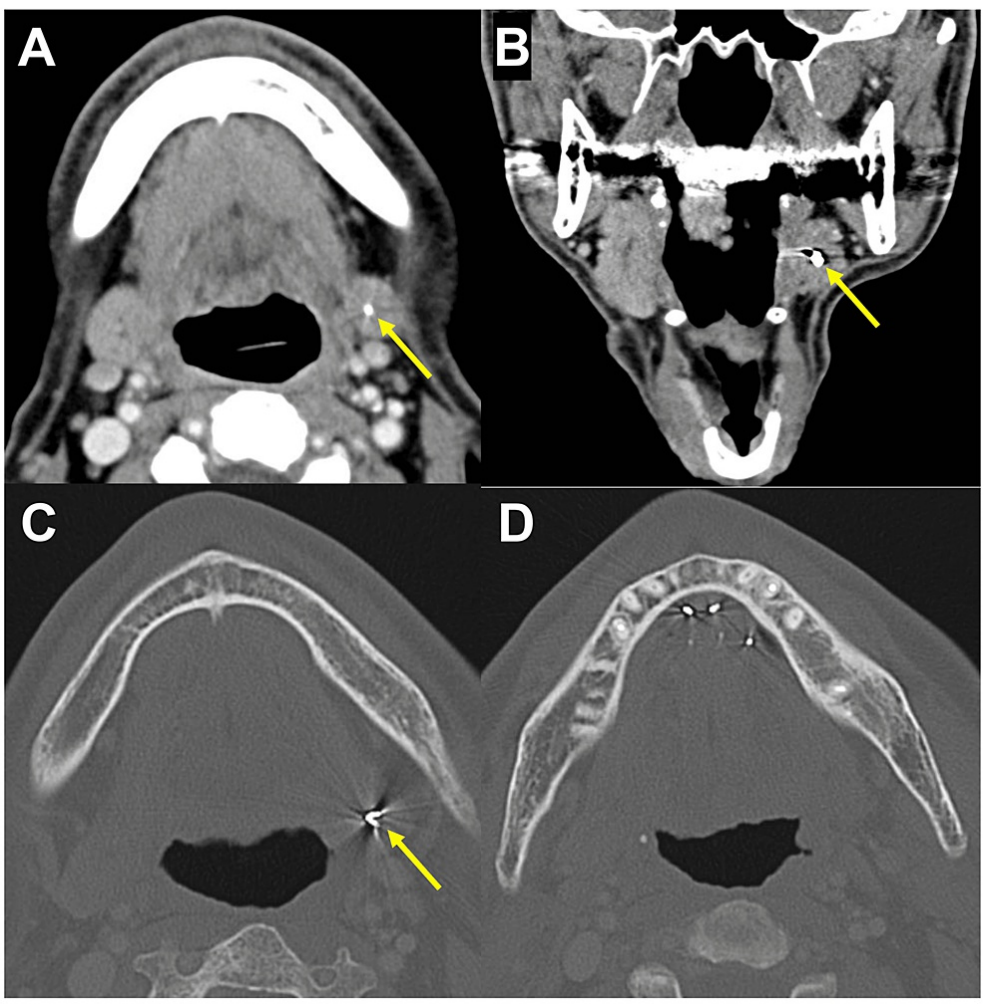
Contrast-enhanced CT 11 months after the implant. Detection of a gold grain in the left submandibular gland in the soft tissue (A, axial; B, coronal) and bone (C, axial) window images (yellow arrows, a gold grain in the submandibular gland). Detection of multiple gold grains in the anterior floor of the mouth in the bone window image (D). CT: Computed tomography
